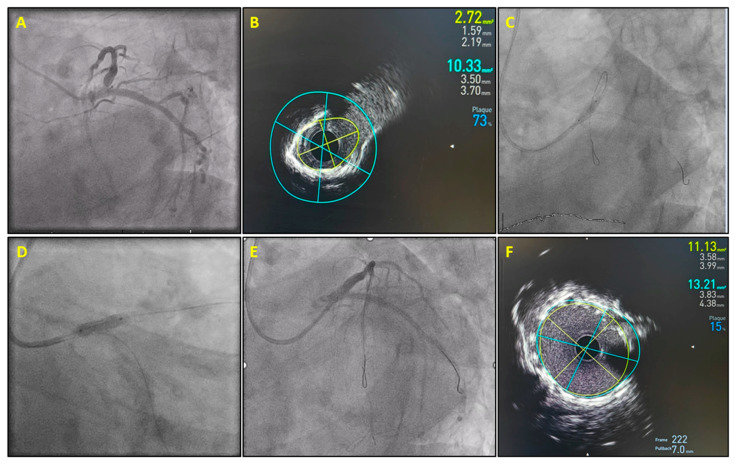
A 73-year-old woman presented complaining of exertional dyspnea. She had undergone left main percutaneous coronary intervention three years earlier. Coronary angiography showed an 80–90% focal stenosis in the ostium of the left anterior descending coronary artery inside the previously implanted stent (A). Intravascular ultrasound (IVUS) showed concentric calcification with underexpansion of the old stent (minimum stent area 2.7 mm^2^) (B). Inflations with a 4.0 mm non-compliant balloon failed to adequately expand the stent. Intravascular lithotripsy using a 4.0 mm balloon modified calcium and allowed subsequent symmetrical non-compliant balloon expansion (C). A 4.0 × 12 mm Megatron (Boston Scientific, Marlborough, MA, USA) drug-eluting stent was implanted (D). Final coronary angiography showed an excellent angiographic result (E), which was confirmed by IVUS imaging (minimum stent area, 9.1 mm^2^) (F).
